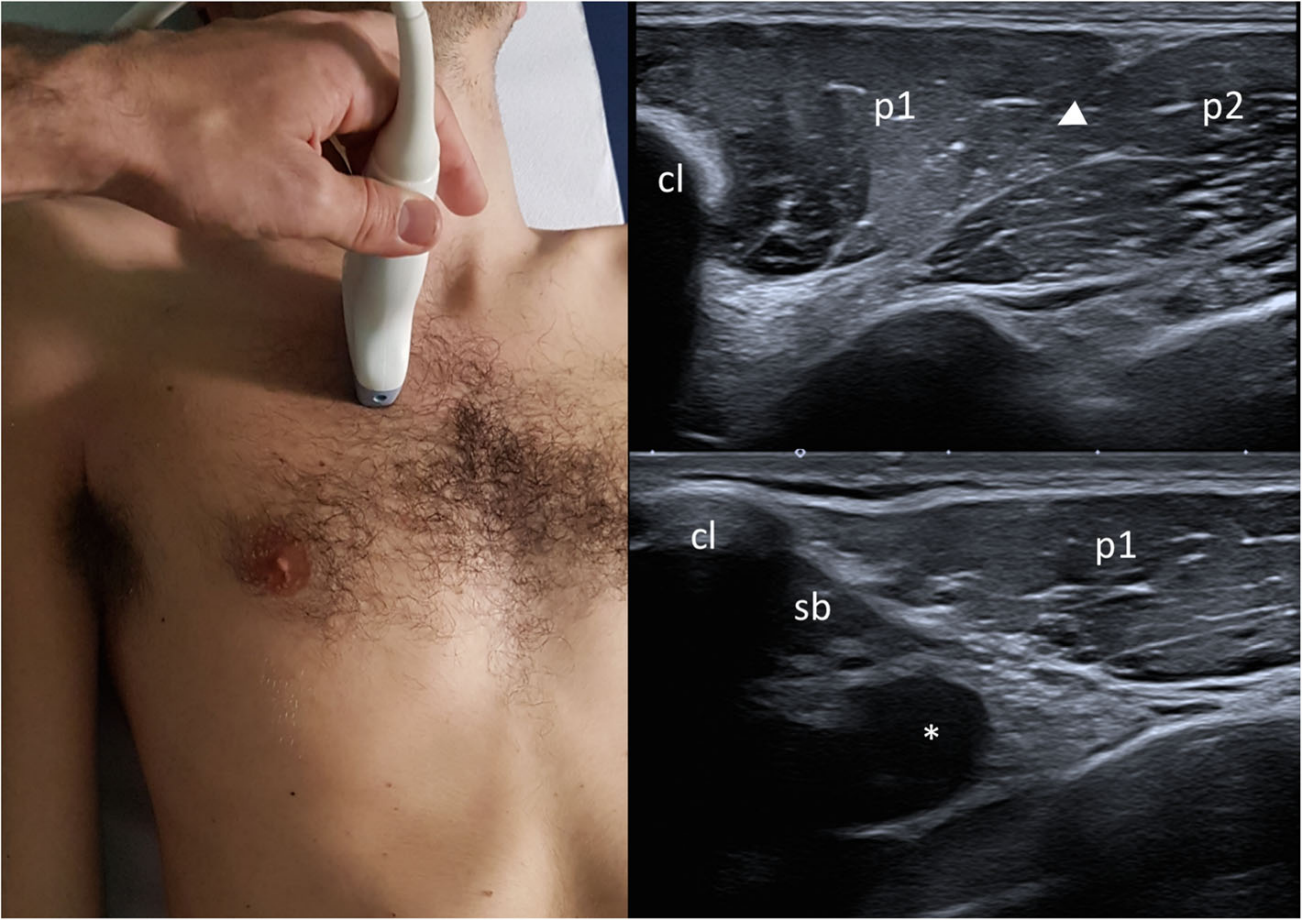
Sagittal parasternal with the upper edge of the probe on the clavicle. Probe on the sagittal axis of the clavicle, immediately lateral of sterno-clavicular joint: at the upper edge of probe there is the clavicle (cl) where clavicular component of pectoral major (p1) takes origin; a cleavage plane (arrow head) with the sterno-costal component (p2) is visible. Starting from the midportion of the clavicle and continuing laterally, the subclavius muscle (sb) is identified underneath the bone, parallel to the long axis of clavicule, crossed below by axillary artery (*) and vein (collapsed in the image) and brachial plexus cord
